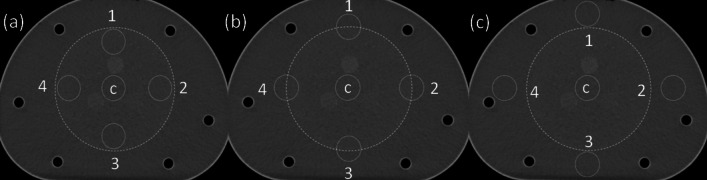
CT value acquisition for image uniformity for (a) background of CT module, (b) boundary between background and purified water, and (c) pure water area. The circles 1–4 represent ROIs 1–4, and the circle “c” represents “ROI-c”
